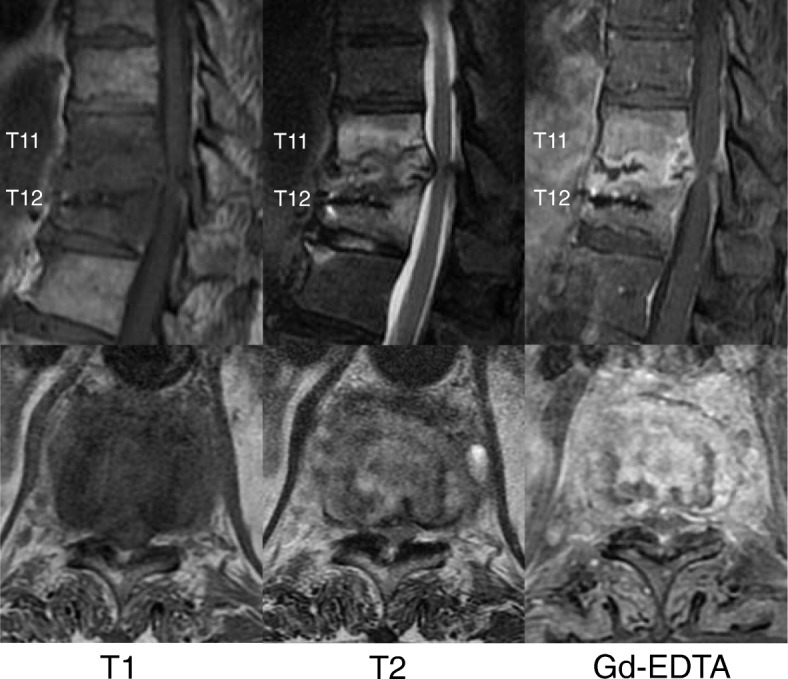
Gadolinium-enhanced T1-weighted magnetic resonance imaging indicated increased signal intensity at T11–T12 vertebral bodies and severe cord compression and epidural abscess at T11–T12 associated with infiltration of soft paravertebral tissues
